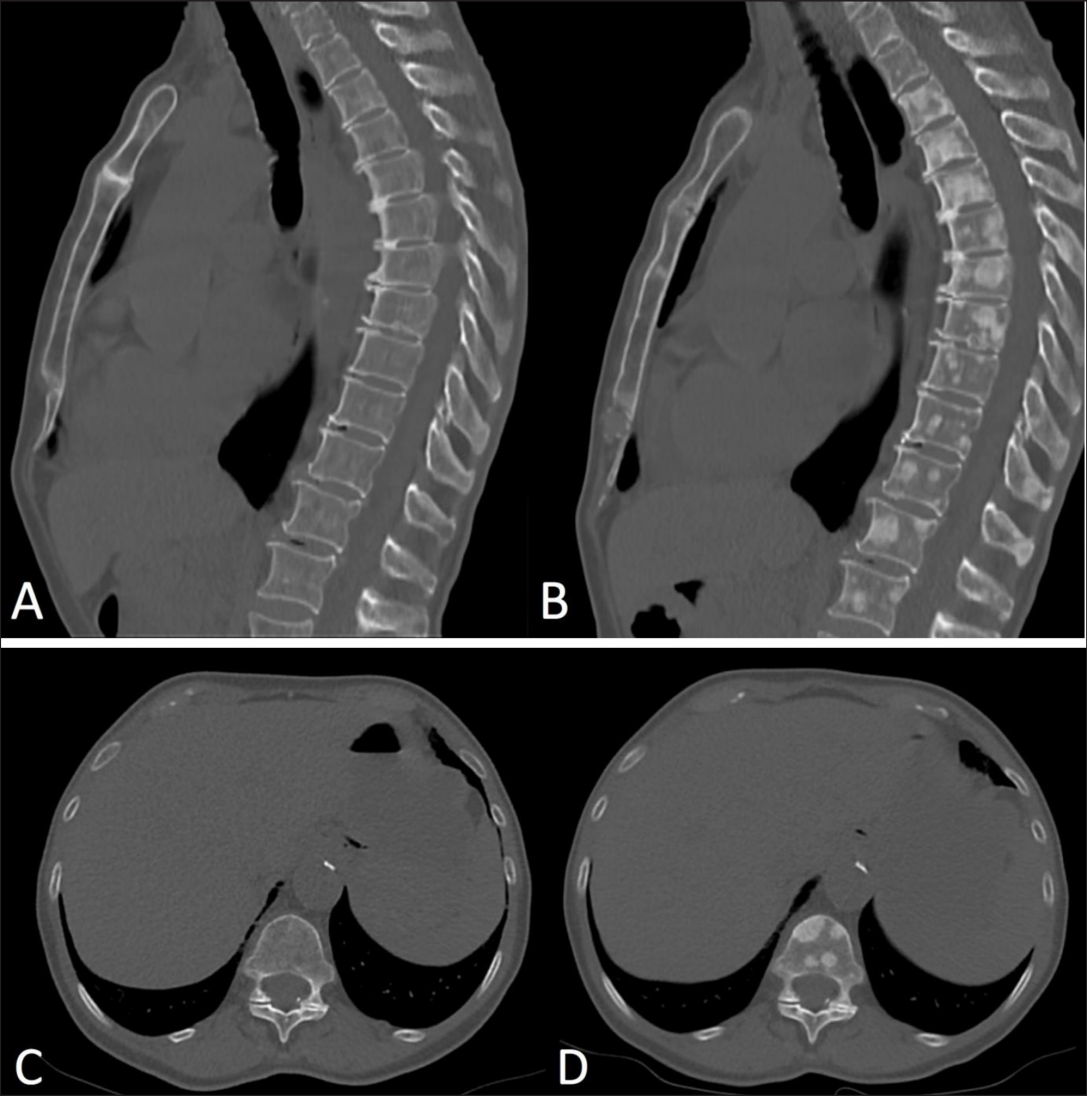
Sagittal and axial CT-images in bone window setting (Figure 1). The baseline study (A, C) shows no focal lytic or blastic bone lesions. First follow-up CT after eight weeks of treatment with erlotinib (B, D) shows numerous new blastic bone lesions in the spine, ribs and sternum. Misinterpretation of these findings as new metastases would classify this patient as progressive cancer disease.
